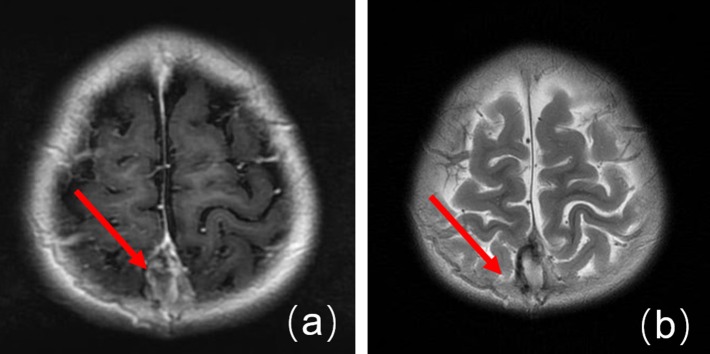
Enhanced MRI before treatment. (a) An MRI image showing a T1WI high-signal in the superior sagittal sinus, plus a slab-like low signal. (b) An MRI image showing a T2WI high-signal in the superior sagittal sinus.
